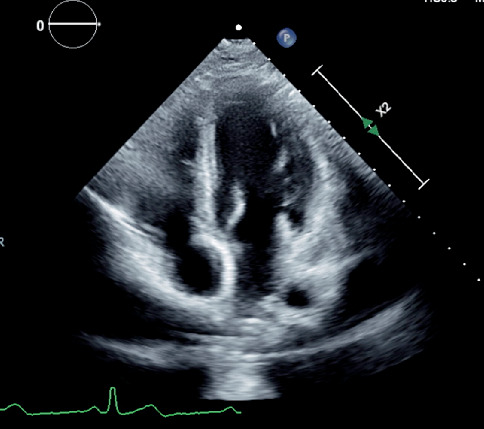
Transthoracic echocardiogram demonstrating the resolution of the previously observed vegetations.
